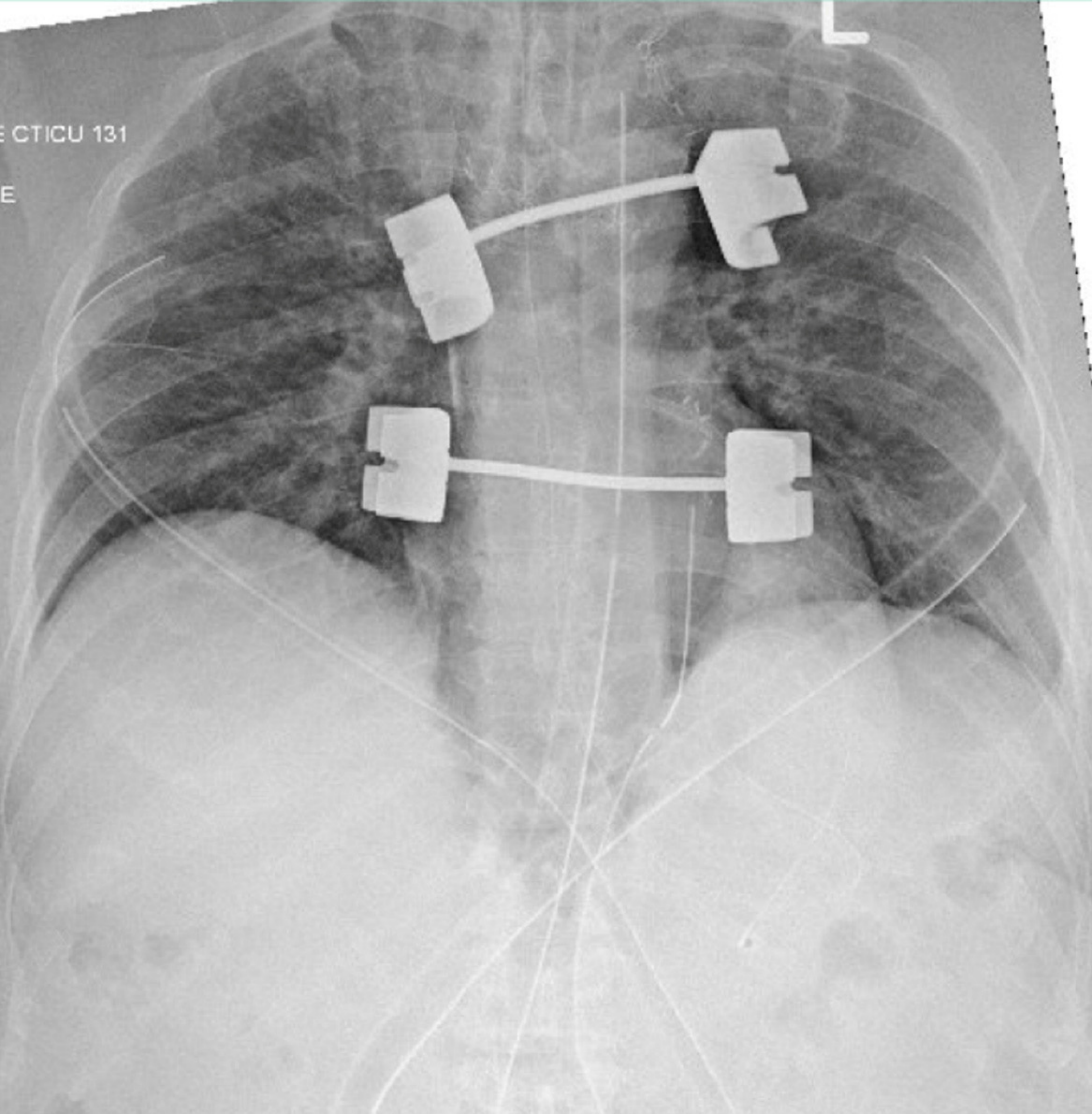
A chest roentgenogram taken in the ICU, showing pulmonary edema as well as sternal stents in situ.
